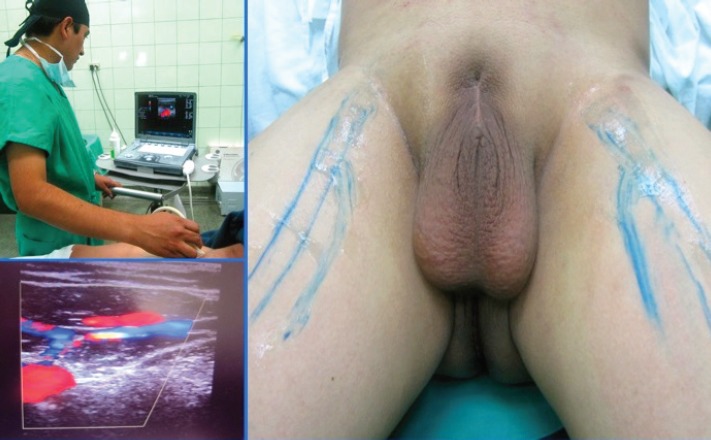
Doppler ultrasound of inguinofemoral region with the location of femoral vessels, the venous arch and saphenous vein, and marking of them on the skin.
